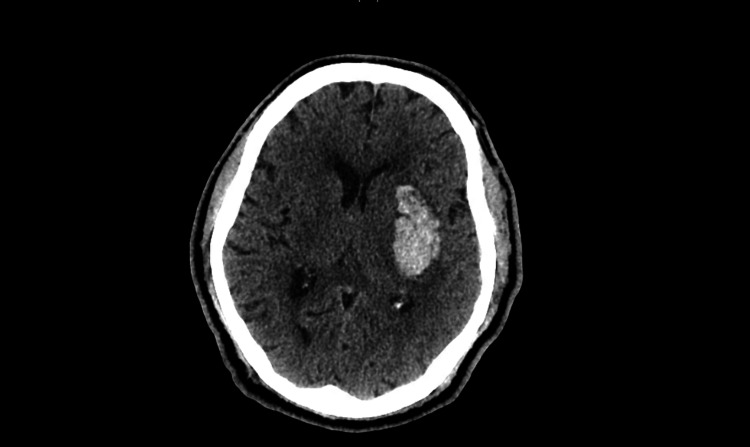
CT head without contrast showing acute left basal ganglia hematoma Acute left basal ganglia hematoma (volume approximately 16.5 mL) with local mass effect and partial effacement left lateral ventricle. Minimal left to right shift of midline at the level of the septum pellucidum.
